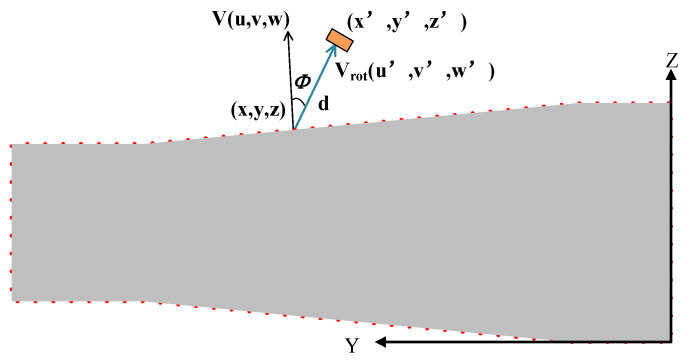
Probe pose calculation based on ultrasonic point cloud.
